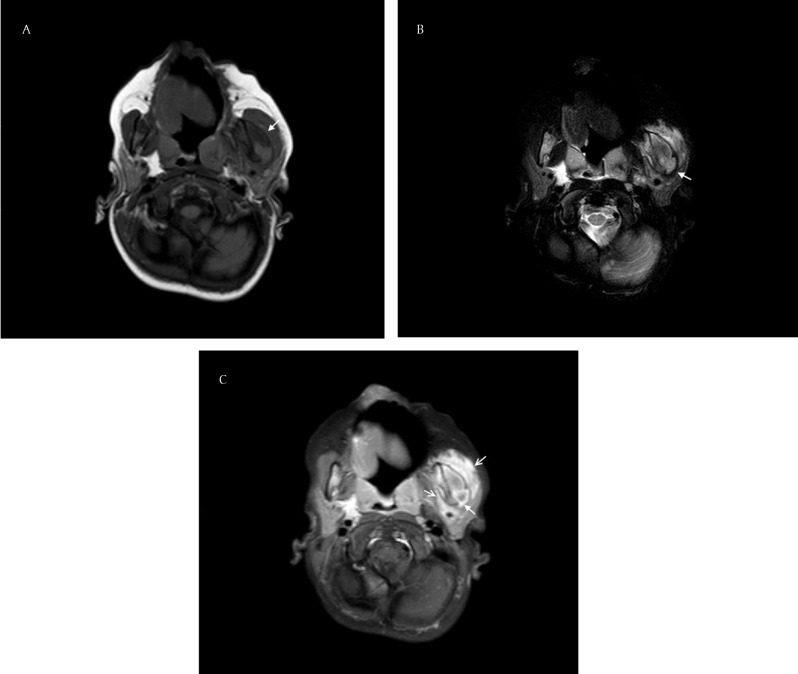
(A) T1-weighted axial image of the mandible demonstrating a bone reaction on the left side of the mandible with low signal (solid white arrow); (B) Fat-suppressed T2-weighted axial image of the mandible showing a bone reaction on the left side of the mandible with high signal (solid white arrow); (C) Gadolinium-enhanced T1-weighted axial image of the mandible, enhancement of Gadolinium is seen in the bone (solid white arrow) and in the adjacent tissues (hollow white arrows).
